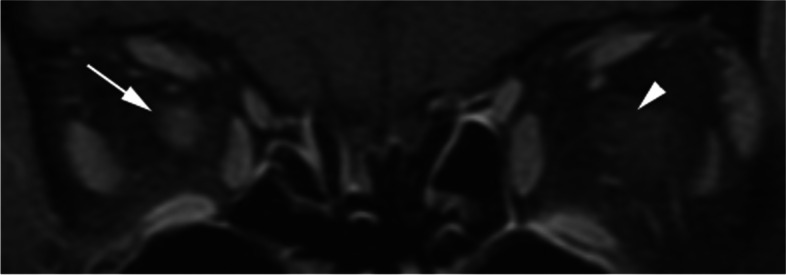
MRI orbits with contrast and fat suppression (coronal view) showing right optic nerve enhancement (arrow), with no enhancement in the left optic nerve (arrowhead)
